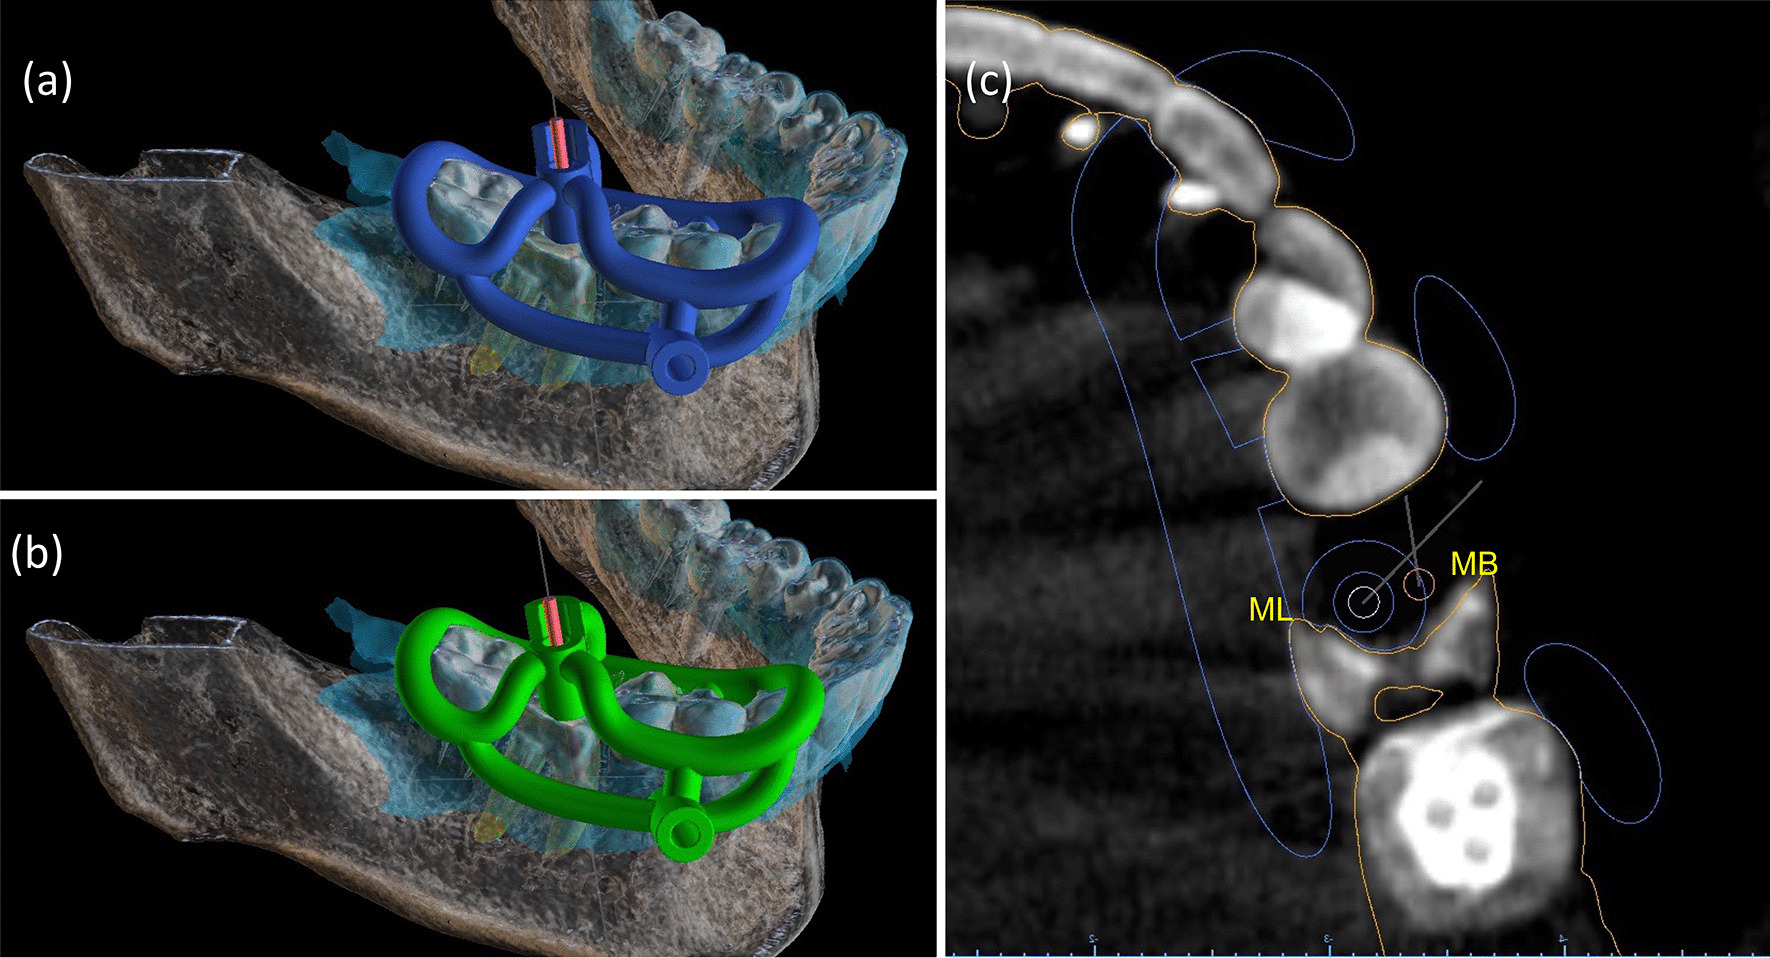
Both MB and ML templates followed an open design concept. The light-blue image represents the scanned area. The virtual ML template with drill and sleeve (a). The virtual MB template with the drill and respective sleeve (b). The resin base of metal sleeves was designed as a half-cylinder to facilitate the insertion of the drill. The top worked as a reference for the drilling length (a and b). The sleeve for the fixation pin had the same position for both templates, avoiding unnecessary bone drilling. CBCT image in the axial plane with virtual drills and sleeve of the ML template (c)
